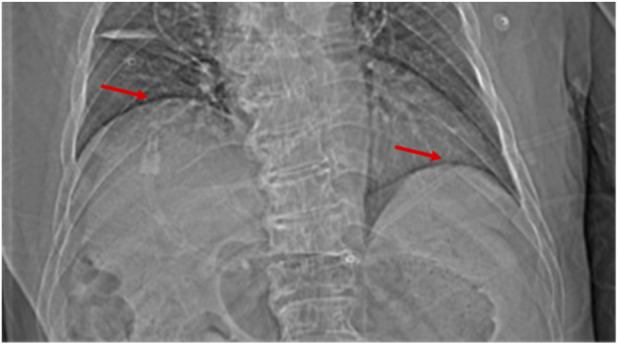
Chest CT (scout view) on postoperative day 22 showing further elevation of the right hemidiaphragm.
